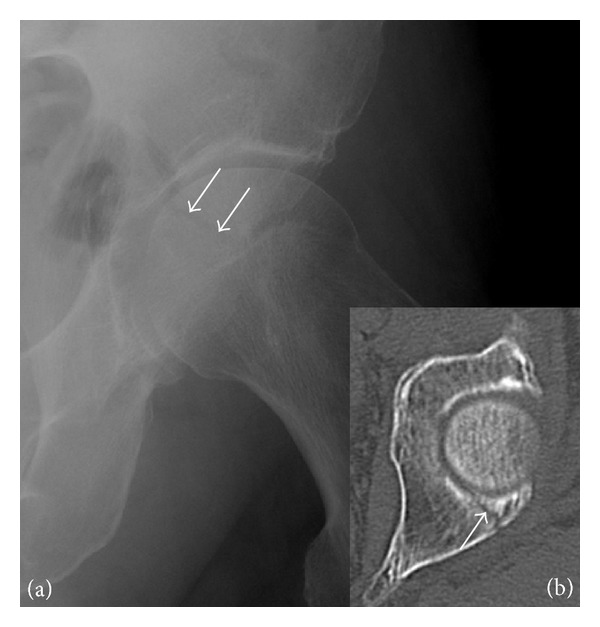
Posterior acetabular fracture in a 49-year-old woman presenting with hip pain after a fall. (a) Anteroposterior radiograph of the left hip shows a radiolucent line through the posterior acetabular wall (arrows). (b) Axial CT confirms the acetabular fracture (arrow).
